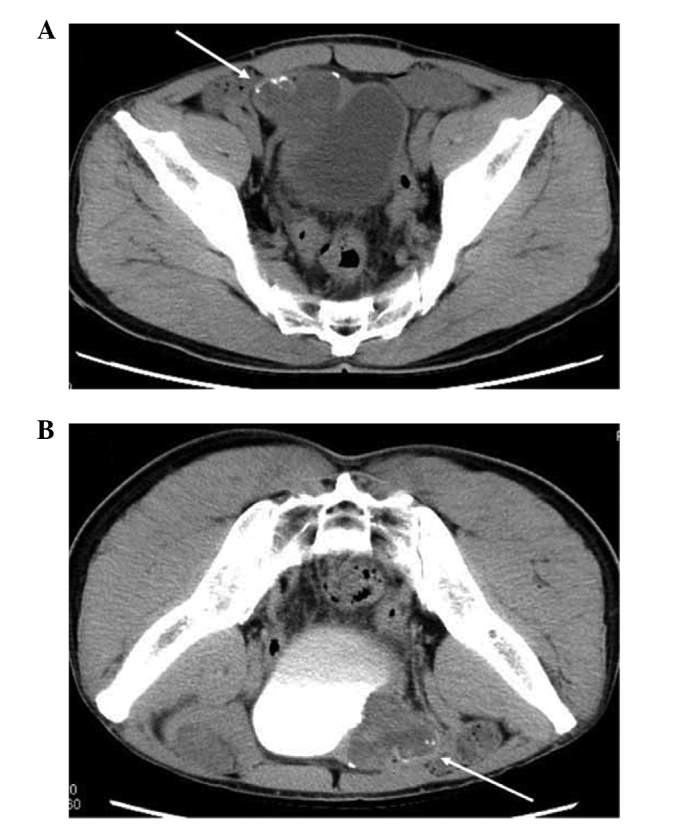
Enhanced abdominal computed tomography scans (transverse images). (A) Plain scan demonstrating a hypodense mass at the level of the right anterior bladder wall with sporadic calcifications in the peripheral area, as indicated by the arrow. (B) In postcontrast imaging (prone position), the mass was moderately and heterogeneously enhanced, as indicated by the arrow.
